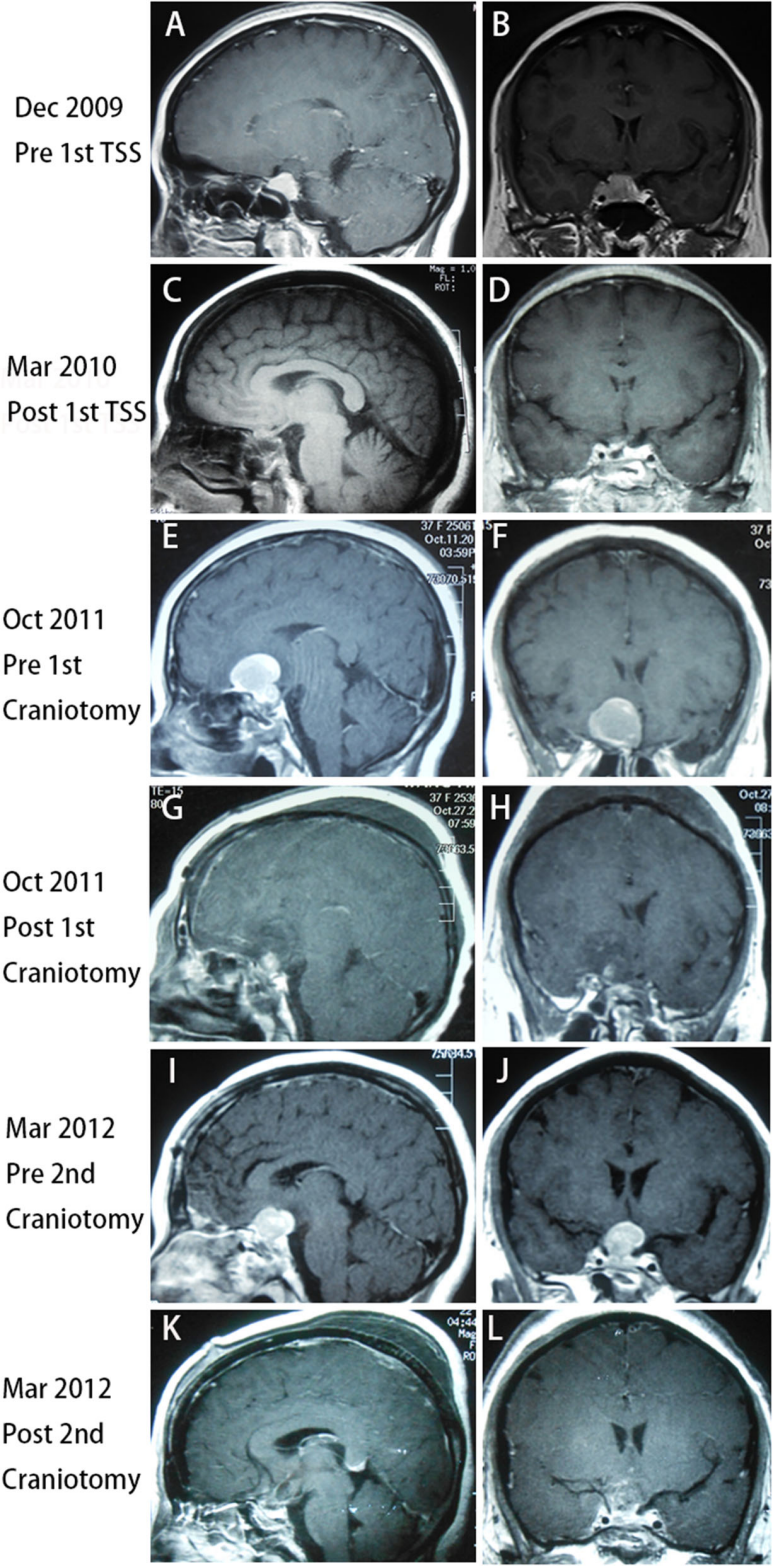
Preoperative sagittal (A) and coronal (B) planes of magnetic resonance imaging (MRI) revealed a pituitary macroadenoma 2.5 cm*2.0 cm that invaded the right cavernous sinus and encased the right internal carotid artery (ICA) completely (Knosp grade 4). (C) and (D) Three months after the first transsphenoidal surgery (TSS), MRI indicated that the tumor was gross totally resected. (E) and (F) Before the first frontal craniotomy, MRI in October 2011 revealed a rapidly enlarged tumor with compression of the optic chiasm. (G) and (H) After the first frontal craniotomy, MRI demonstrated that the tumor was subtotally removed. (I) and (J) Before the second craniotomy, MRI in March 2012 indicated a rapidly growing tumor with compression of the optic chiasm and invasion into the third ventricle. (K) and (L) After the second frontal craniotomy, the tumor was subtotal resected
